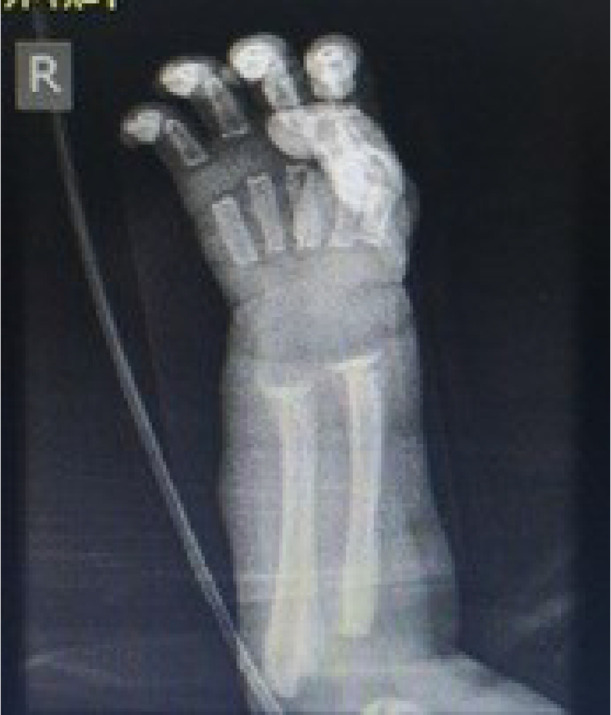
Wrist x-ray of neonatal for diagnosis prematurity osteoporosis.
